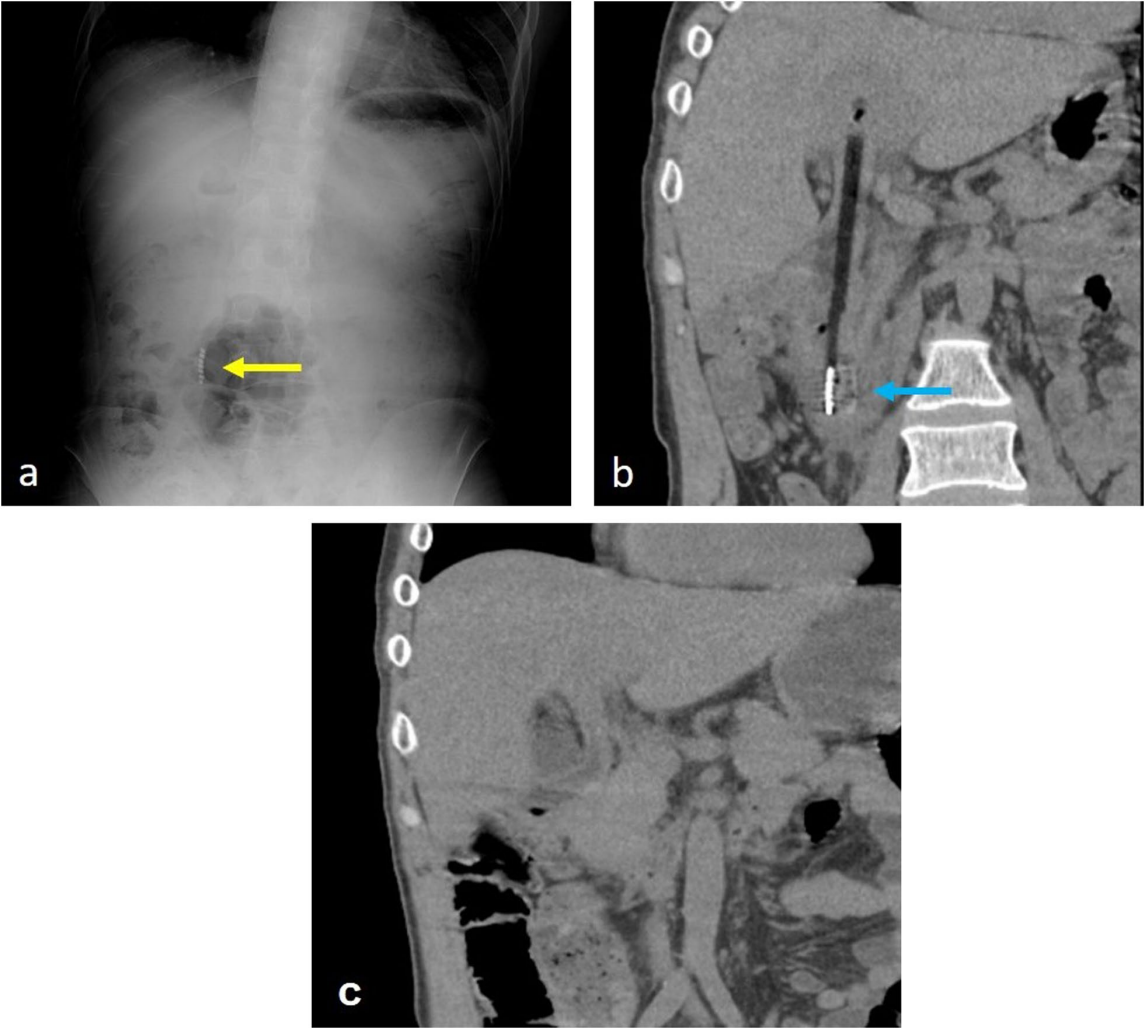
a A plain abdominal X-ray suggesting a foreign body (yellow arrow). b A computed tomography (CT) scan revealing a toothbrush in the duodenum (blue arrow). c A postoperative CT showing no changes in the liver
